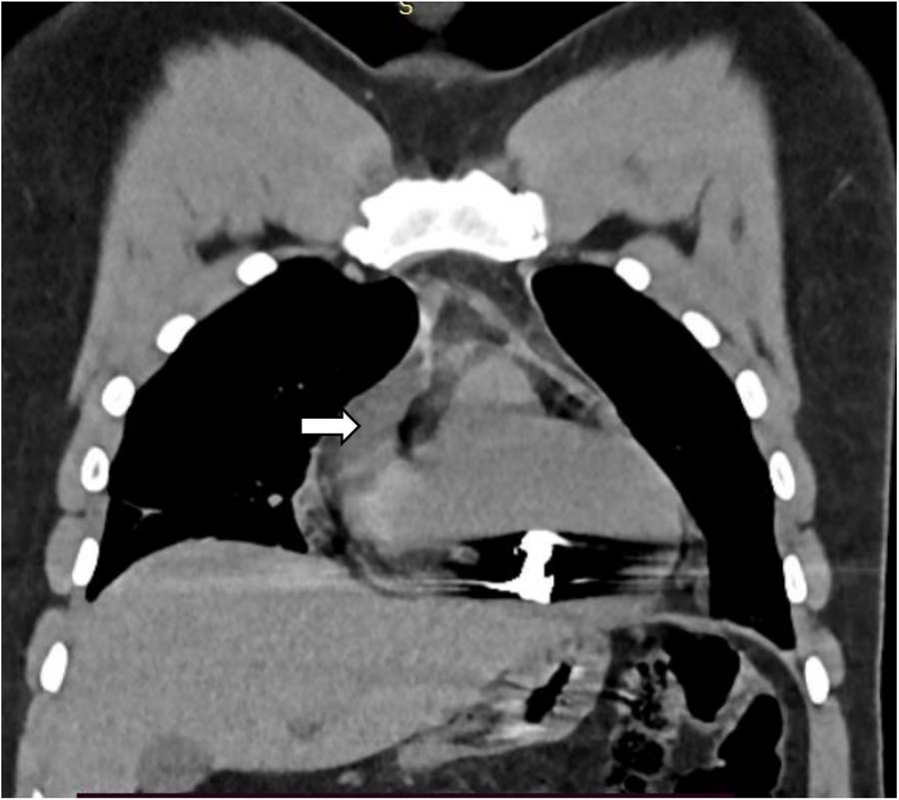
Coronal CT image showing foreign body with in the myocardium of the right ventricle (white arrow).
